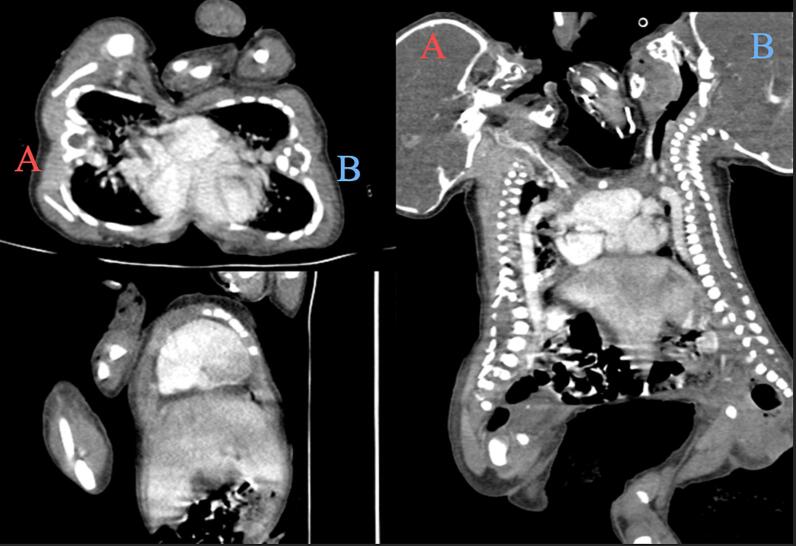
A contrasted CT scan in axial, coronal and sagittal views with twin A on the left and twin B on the right. It shows conjoined twins with fused chest and abdomen. The pericardium and heart are fused. There is enhancement of twin B’s heart and its vessels. The heart and liver are fused. Twin A has larger cardiac chambers than twin B.
